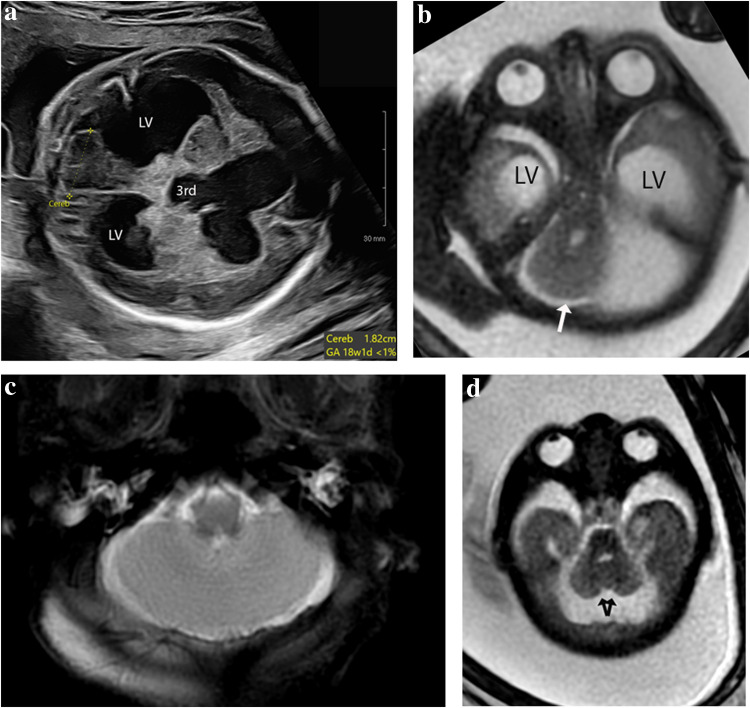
Rhombencephalosynapsis (RES). Prenatal ultrasound (a) at 26 3/7 weeks shows a transverse cerebellar diameter less than the first percentile for gestation. Severe supratentorial ventriculomegaly is also appreciable (*LV*, lateral ventricle; *3rd*, third ventricle). Subsequent fetal MRI performed at 32 2/7 weeks shows findings of RES. Axial T2 through the posterior fossa (b) demonstrates an abnormal contour of the dorsal cerebellum, with a continuous convex contour (*arrow*) and obvious severe supratentorial ventriculomegaly secondary to aqueductal stenosis. Postnatal brain MRI (c) axial T2 image through the posterior fossa shows the abnormal union of the two cerebellar hemispheres. d Normal appearance of the cerebellar vermis and hemispheres in the axial plane in a different fetus imaged at 31 weeks. Note normal clefts (*arrows*) between the vermis and hemispheres to compare to that seen with RES (b)
